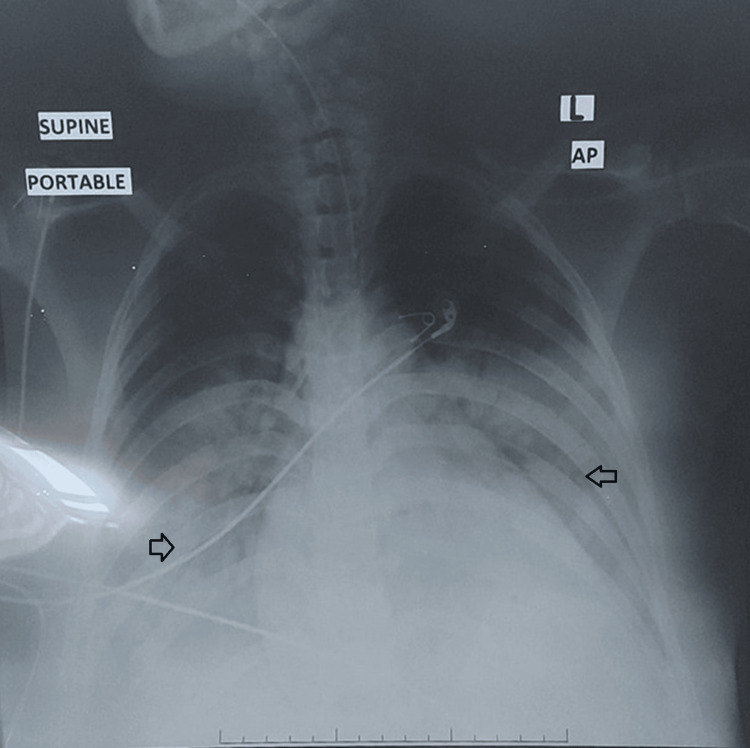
Chest X-ray showed bilateral infiltrates, a normal cardiothoracic ratio, and an endotracheal tube in the correct position.
